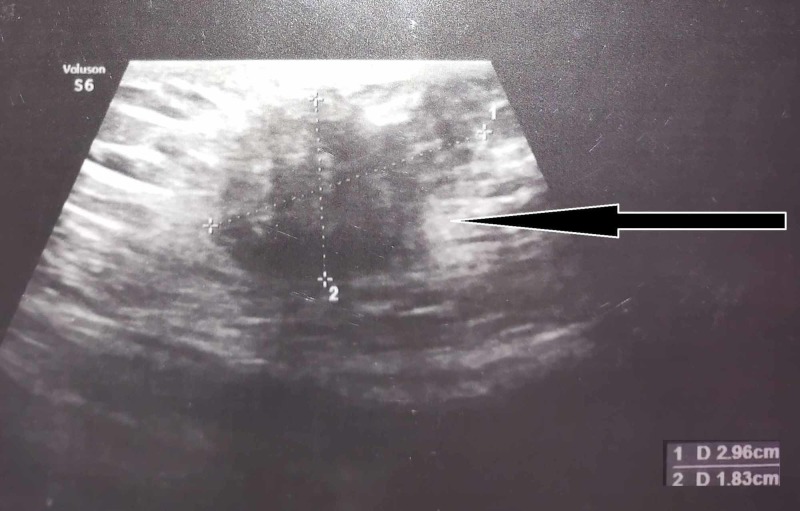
Ultrasound of the abdomen showing a hypoechoic mass (endometrioma) below the cesarean scar.
